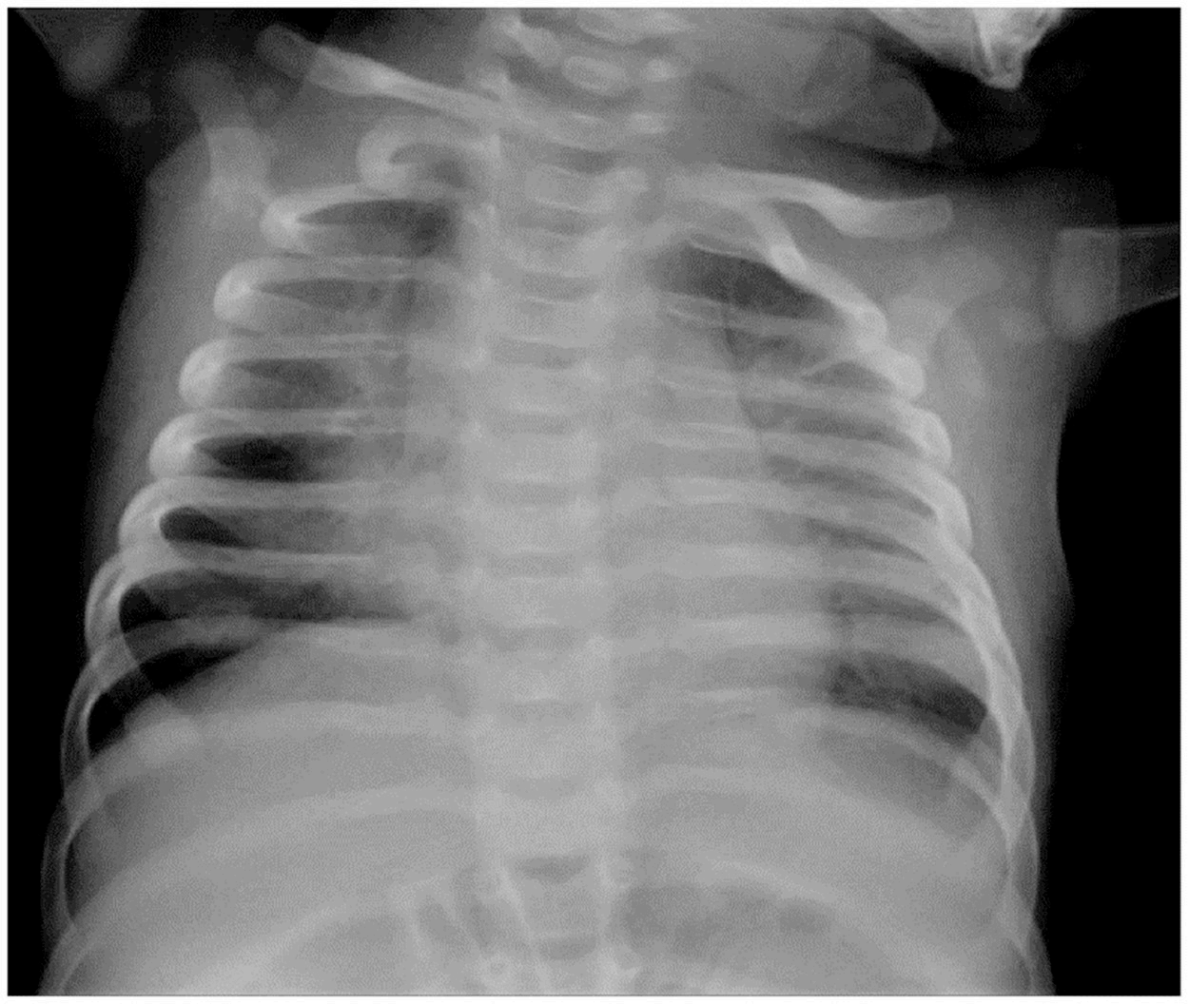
Patient 8 chest X-ray at 4 months.
